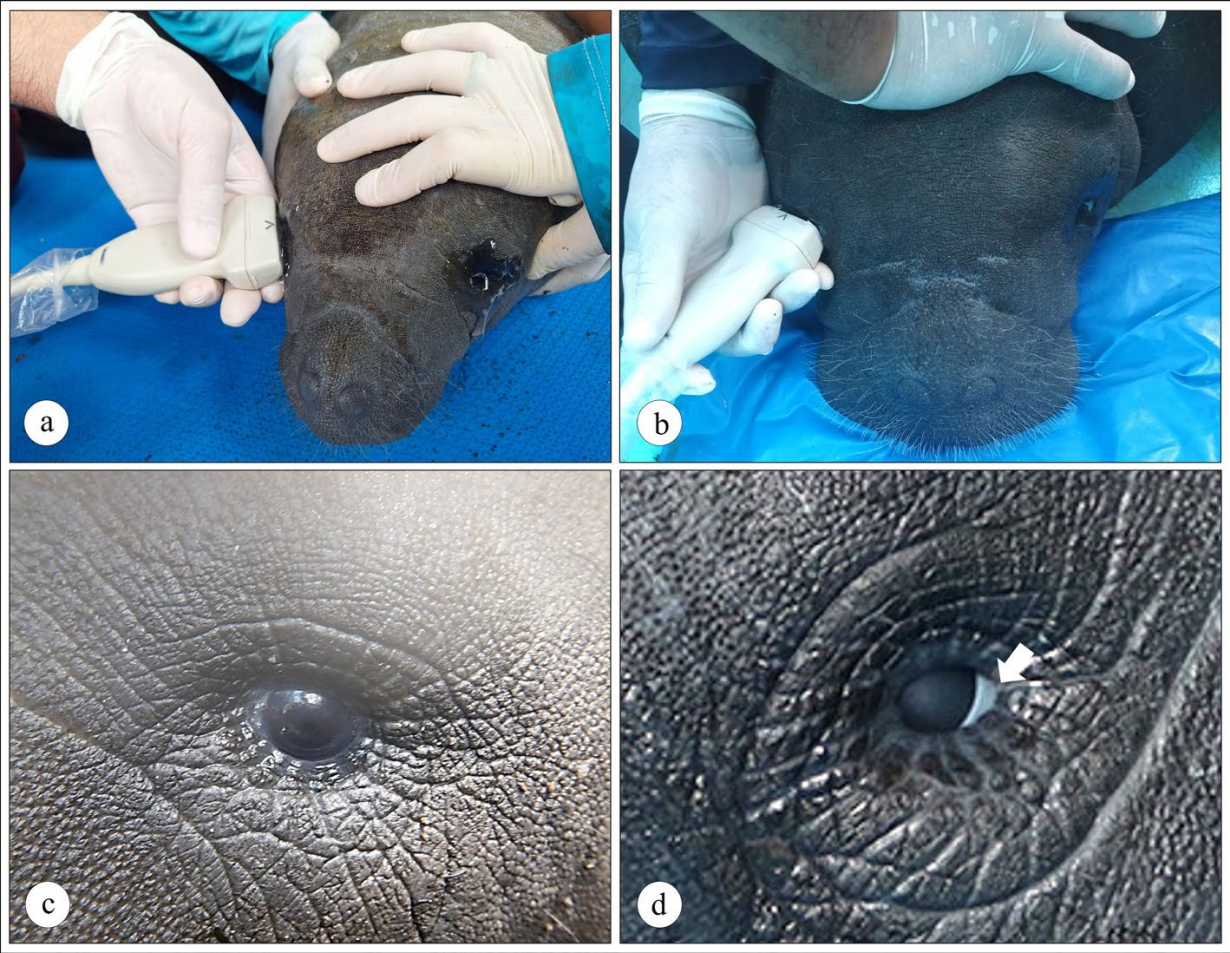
B‐mode ocular ultrasound of Greater Caribbean manatees (
